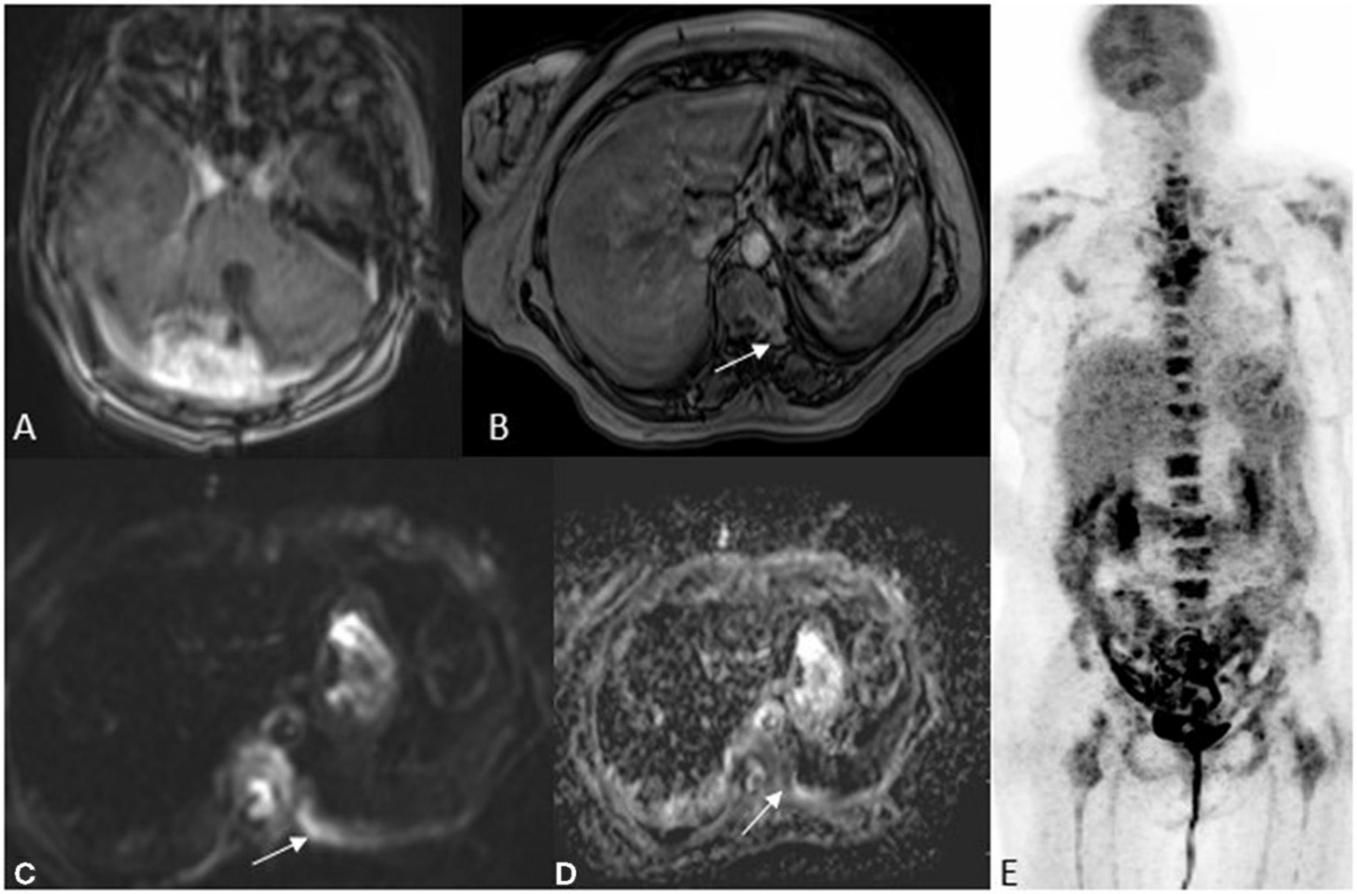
A 52 year old female with imbalance, MRI FDG PET axial brain images T1 post-contrast (A) image showed an enhancing lesion in the right cerebellum. Whole-body MRI with fast sequences at axial hepatic sections (B) VIBE fat sat post-contrast (C) DWI b800 (D) ADC and NAC PET (E) showing an area of metastases to the vertebral body as an area of restricted diffusion and post-contrast enhancement. HPE was suggestive of Pilocytic astrocytoma with metastasis.
